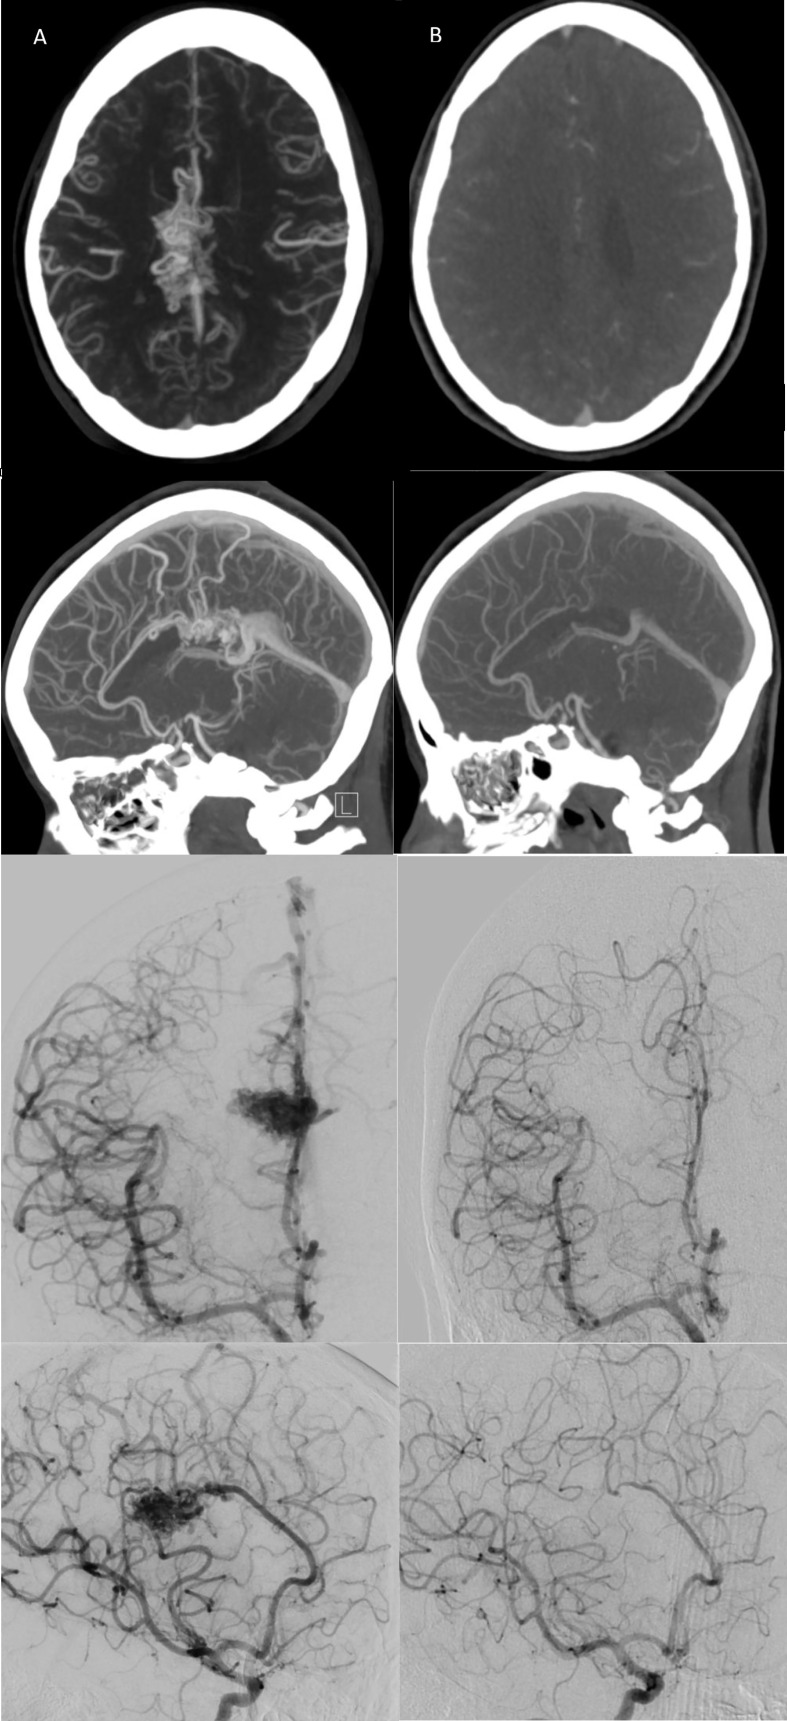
(A) Pre-radiosurgery, and (B) post-radiosurgery axial/sagittal CT angiogram and coronal/sagittal right ICA injection conventional angiograms for a representative case with follow-up images taken at 3 years post-CyberKnife treatment demonstrating complete AVM nidal obliteration with no residual draining vein(s).
